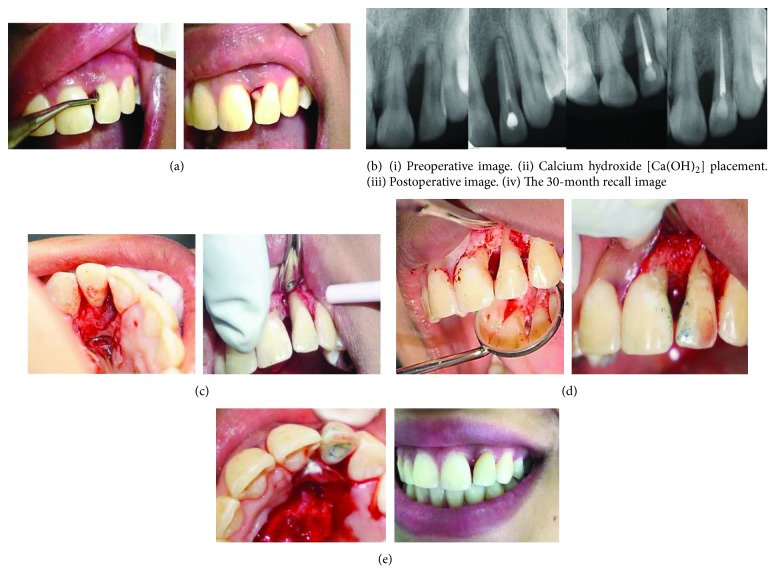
(a) A deep periodontal pocket and drainage of pus through the gingival sulcus are visible. (b) Radiographs of tooth #22 exhibit (i) a large lateral radiolucency on the mesial tooth surface extending from the bone crest to the root apex, (ii) calcium hydroxide [Ca(OH)2] placement, (iii) root canal obturation, and (iv) bone healing. (c) Surgical exposure of tooth #22 shows calculus accumulation and granulation tissue mesially with the absence of buccal bone. (d) Removal of calculus and granulation tissues and placement of the bone graft. (e) Placement of the barrier membrane; the 30-month recall photograph shows normal gingival tissue.
